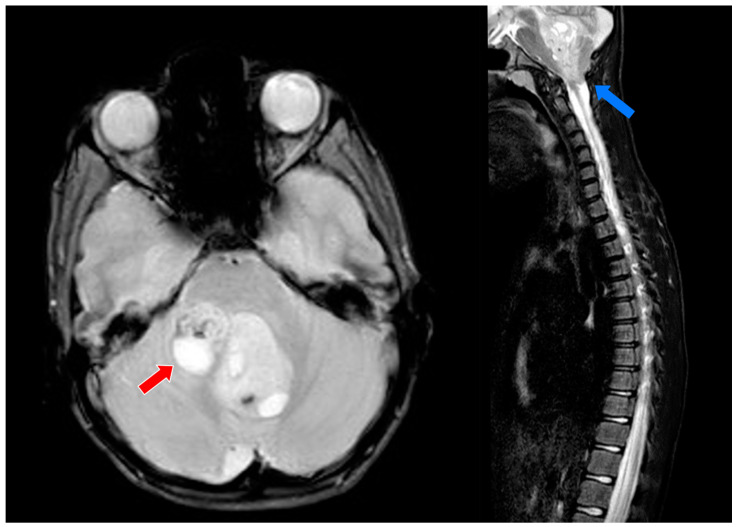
The MRI findings at the time of diagnosis of medulloblastoma in the young patient. The exam revealed a large, lobulated lesion in the right posterior cerebral fossa, appearing hypo-intense on T1-weighted imaging and hyper-intense on T2-weighted imaging, with heterogeneous gadolinium enhancement (red arrow). There was spinal cord compression at the C1 level (blue arrow), obliteration of the fourth ventricle, and cerebellar tonsillar herniation into the foramen magnum. Additionally, diffuse leptomeningeal dissemination was observed, extending ventrally and rostrally from C5 to T2 and T5 to T9 levels.
